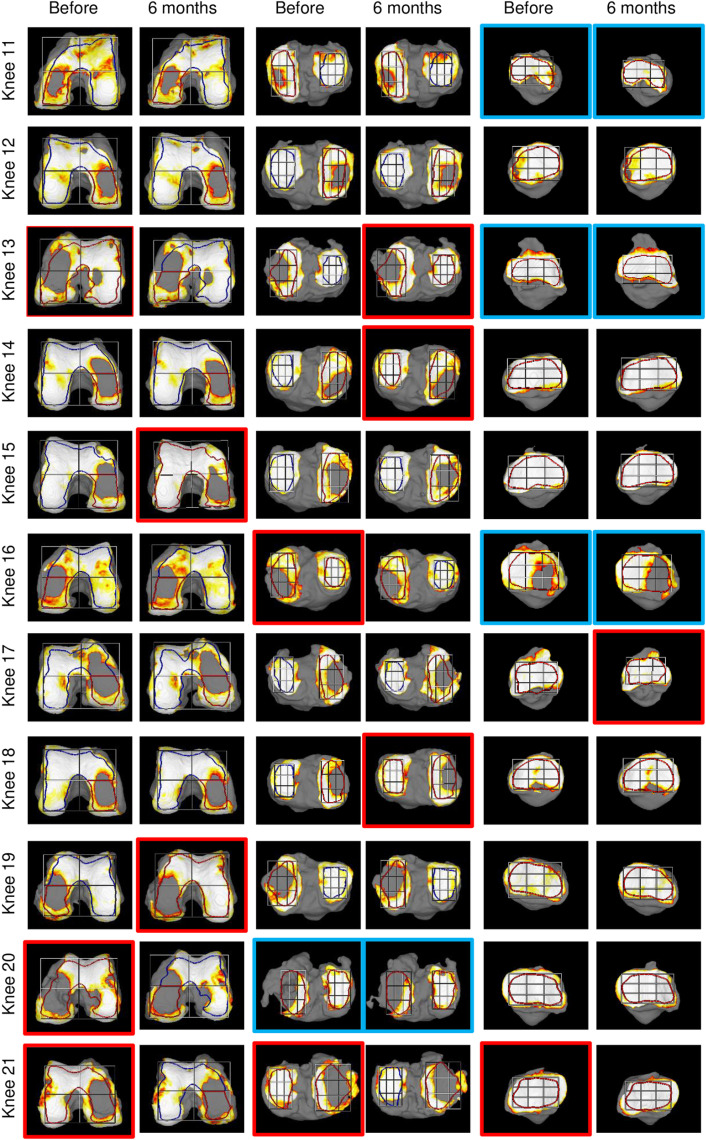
Reconstructed 3D MRI for knee cartilage and ROIs in Knee 11-21, representing the second half of the complete series. All 21 knees (across Fig 3 and Fig 4) are arranged in descending order of PMF cartilage thickness. Red frames indicate inappropriate automatic ROI settings requiring copying, while blue frames show cases where ROI settings for two areas were inappropriate, requiring manual setting of one area and copying to the other.
